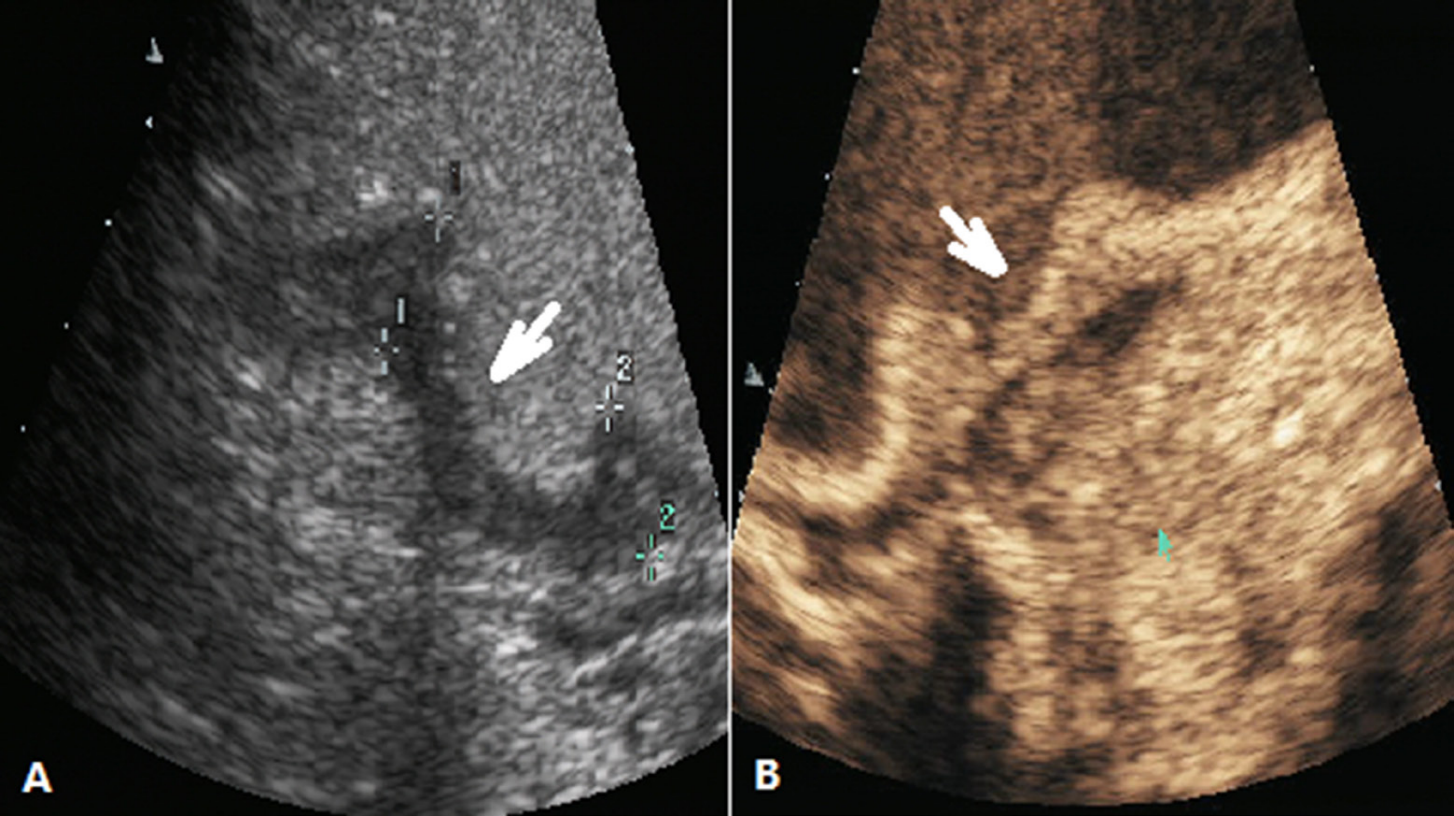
DCEUS images of a case classified as Borrmann type II (A) Ultrasonography showed the ulcerating lesion surrounded by elevated borders (arrow) after the stamoch was filled with UOCA, (B) showed the lesion(arrow) was enhanced after administration of Sonovue.
